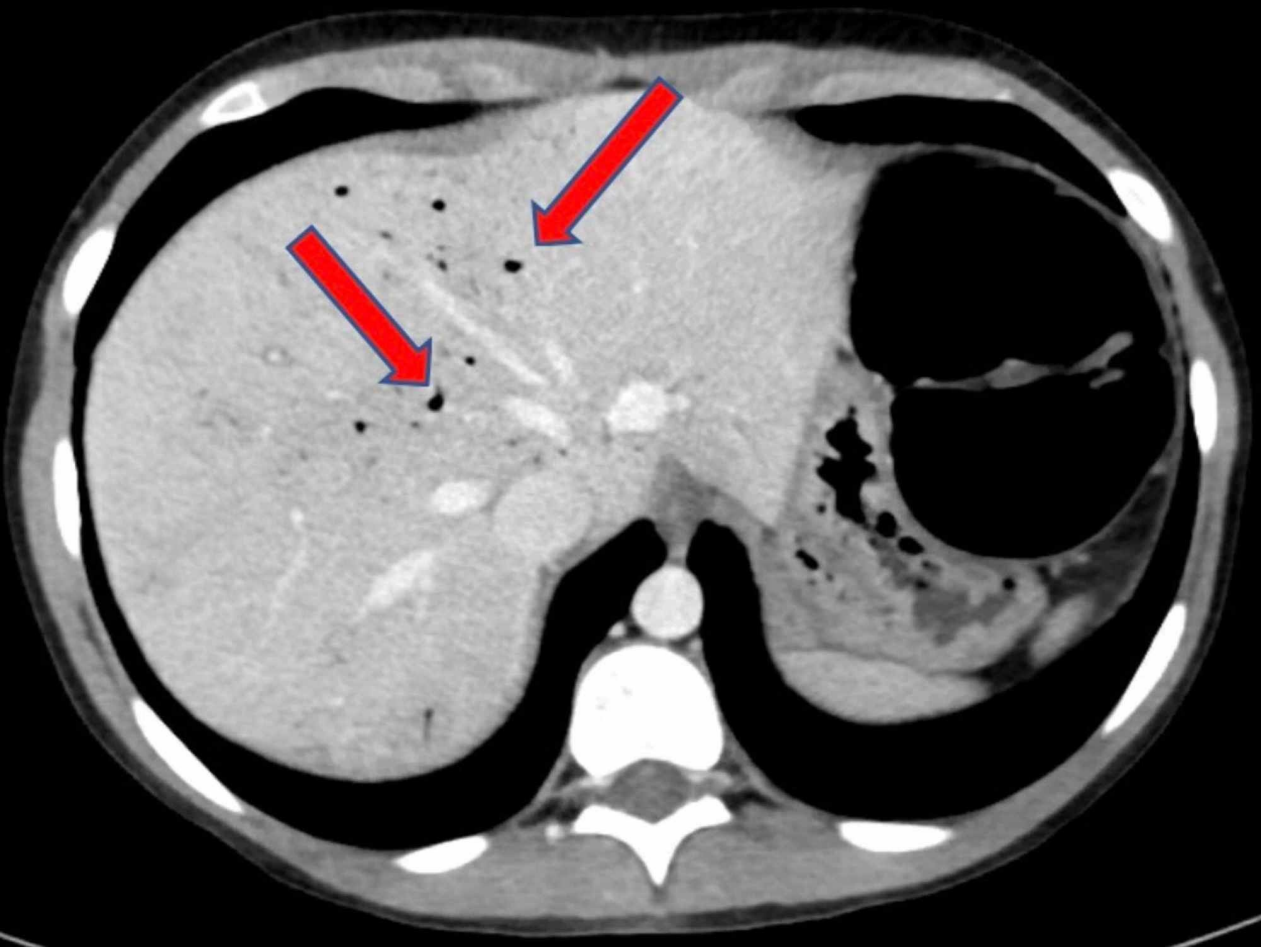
Transverse view CT scan of the abdomen showing marked hepatic venous portal gas
